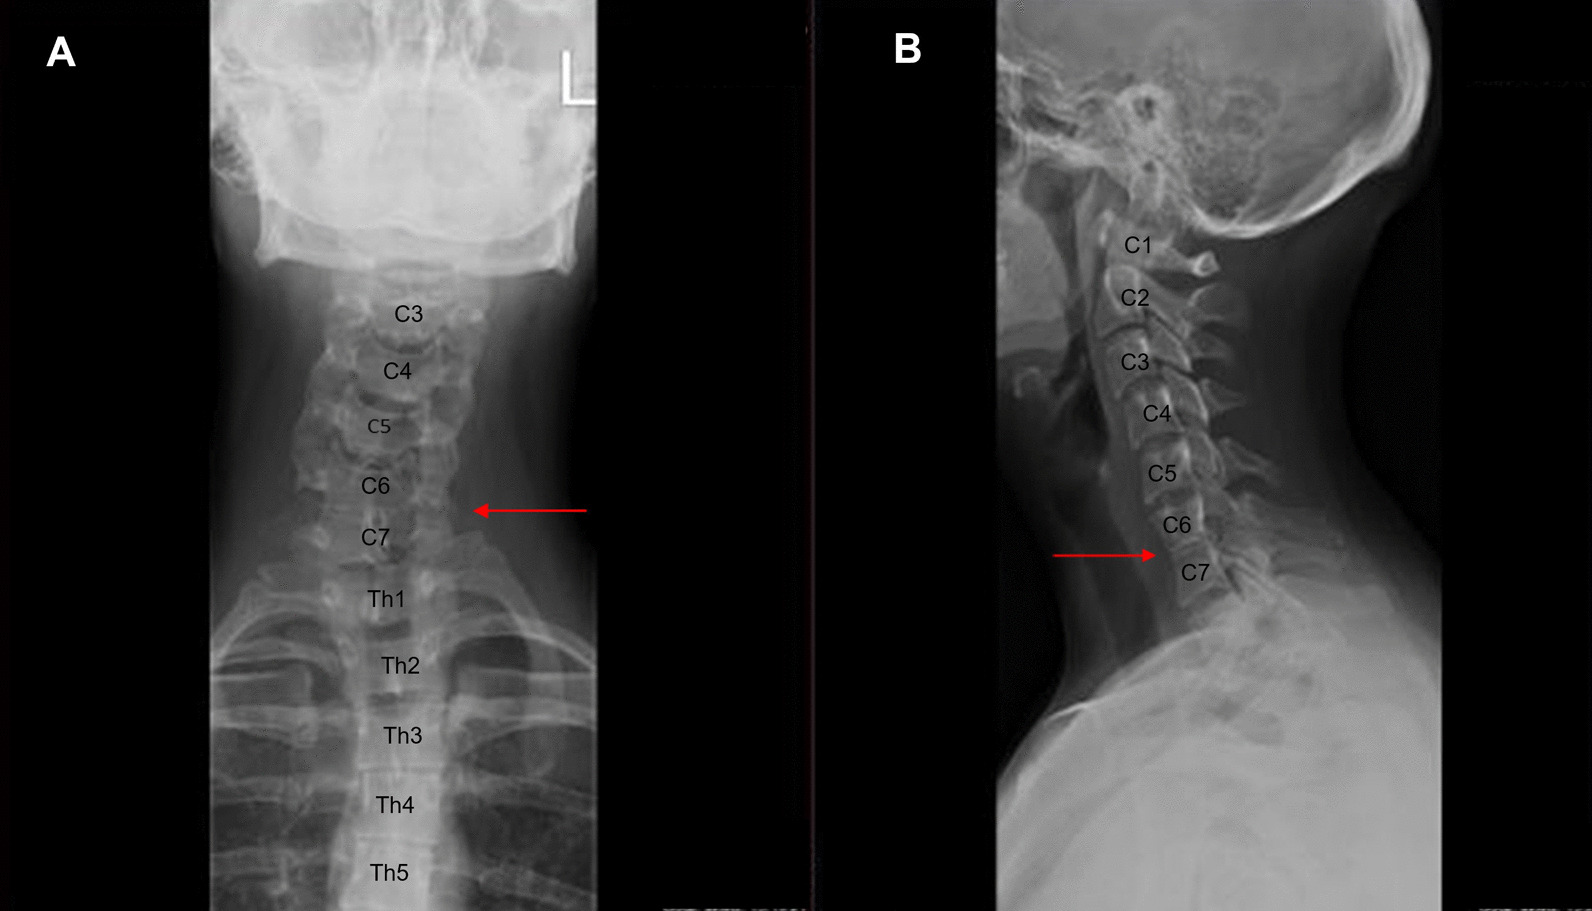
Anteroposterior (A) and lateral (B) cervical spine radiographs showing vertebrae fusion at C6-C7 in a patient with Klippel–Feil syndrome
